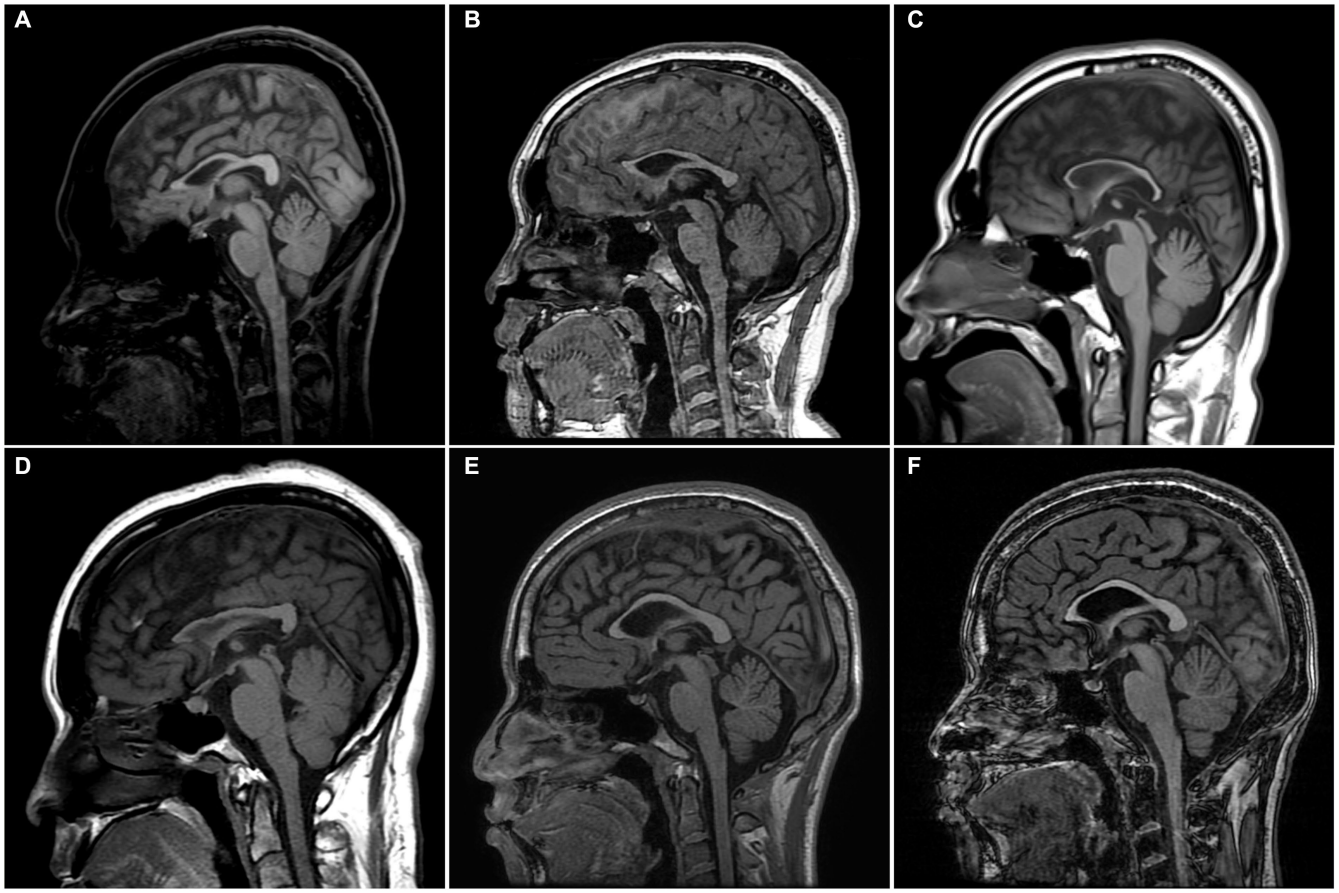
Sagittal T1-weight MR images of the enrolled patients. (A) SN-1, 18 years of age, has focal thinning of region 3 in the corpus callosum. (B) SN-2, 17 years of age, has thinning of regions 3 and 4. (C) SN-3, 22 years of age, has thinning of the entire corpus callosum involving regions 1–7. (D) SN-4, 41 years of age, has thinning of the anterior half of the corpus callosum involving regions 1–4. (E,F) SN-5 and SN-6, 21-year-old identical twins, have focal thinning of region 3. The corpus callosum was divided into seven regions according to Witelson’s guidelines. SN, subject number.
